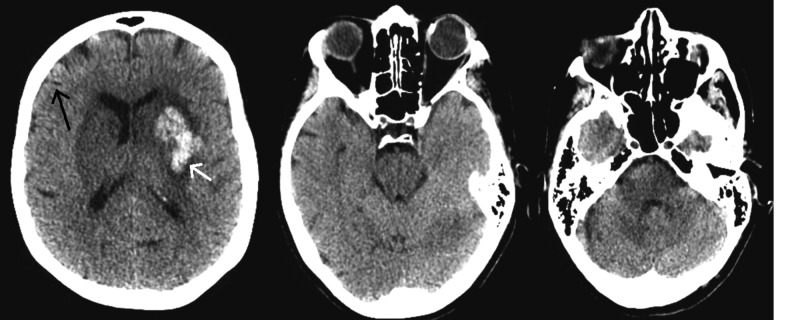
Computed tomography of the head done on postoperative day 8 Cerebral edema improves as gyri again become apparent (black arrow). New left basal ganglia hemorrhage (white arrow)
